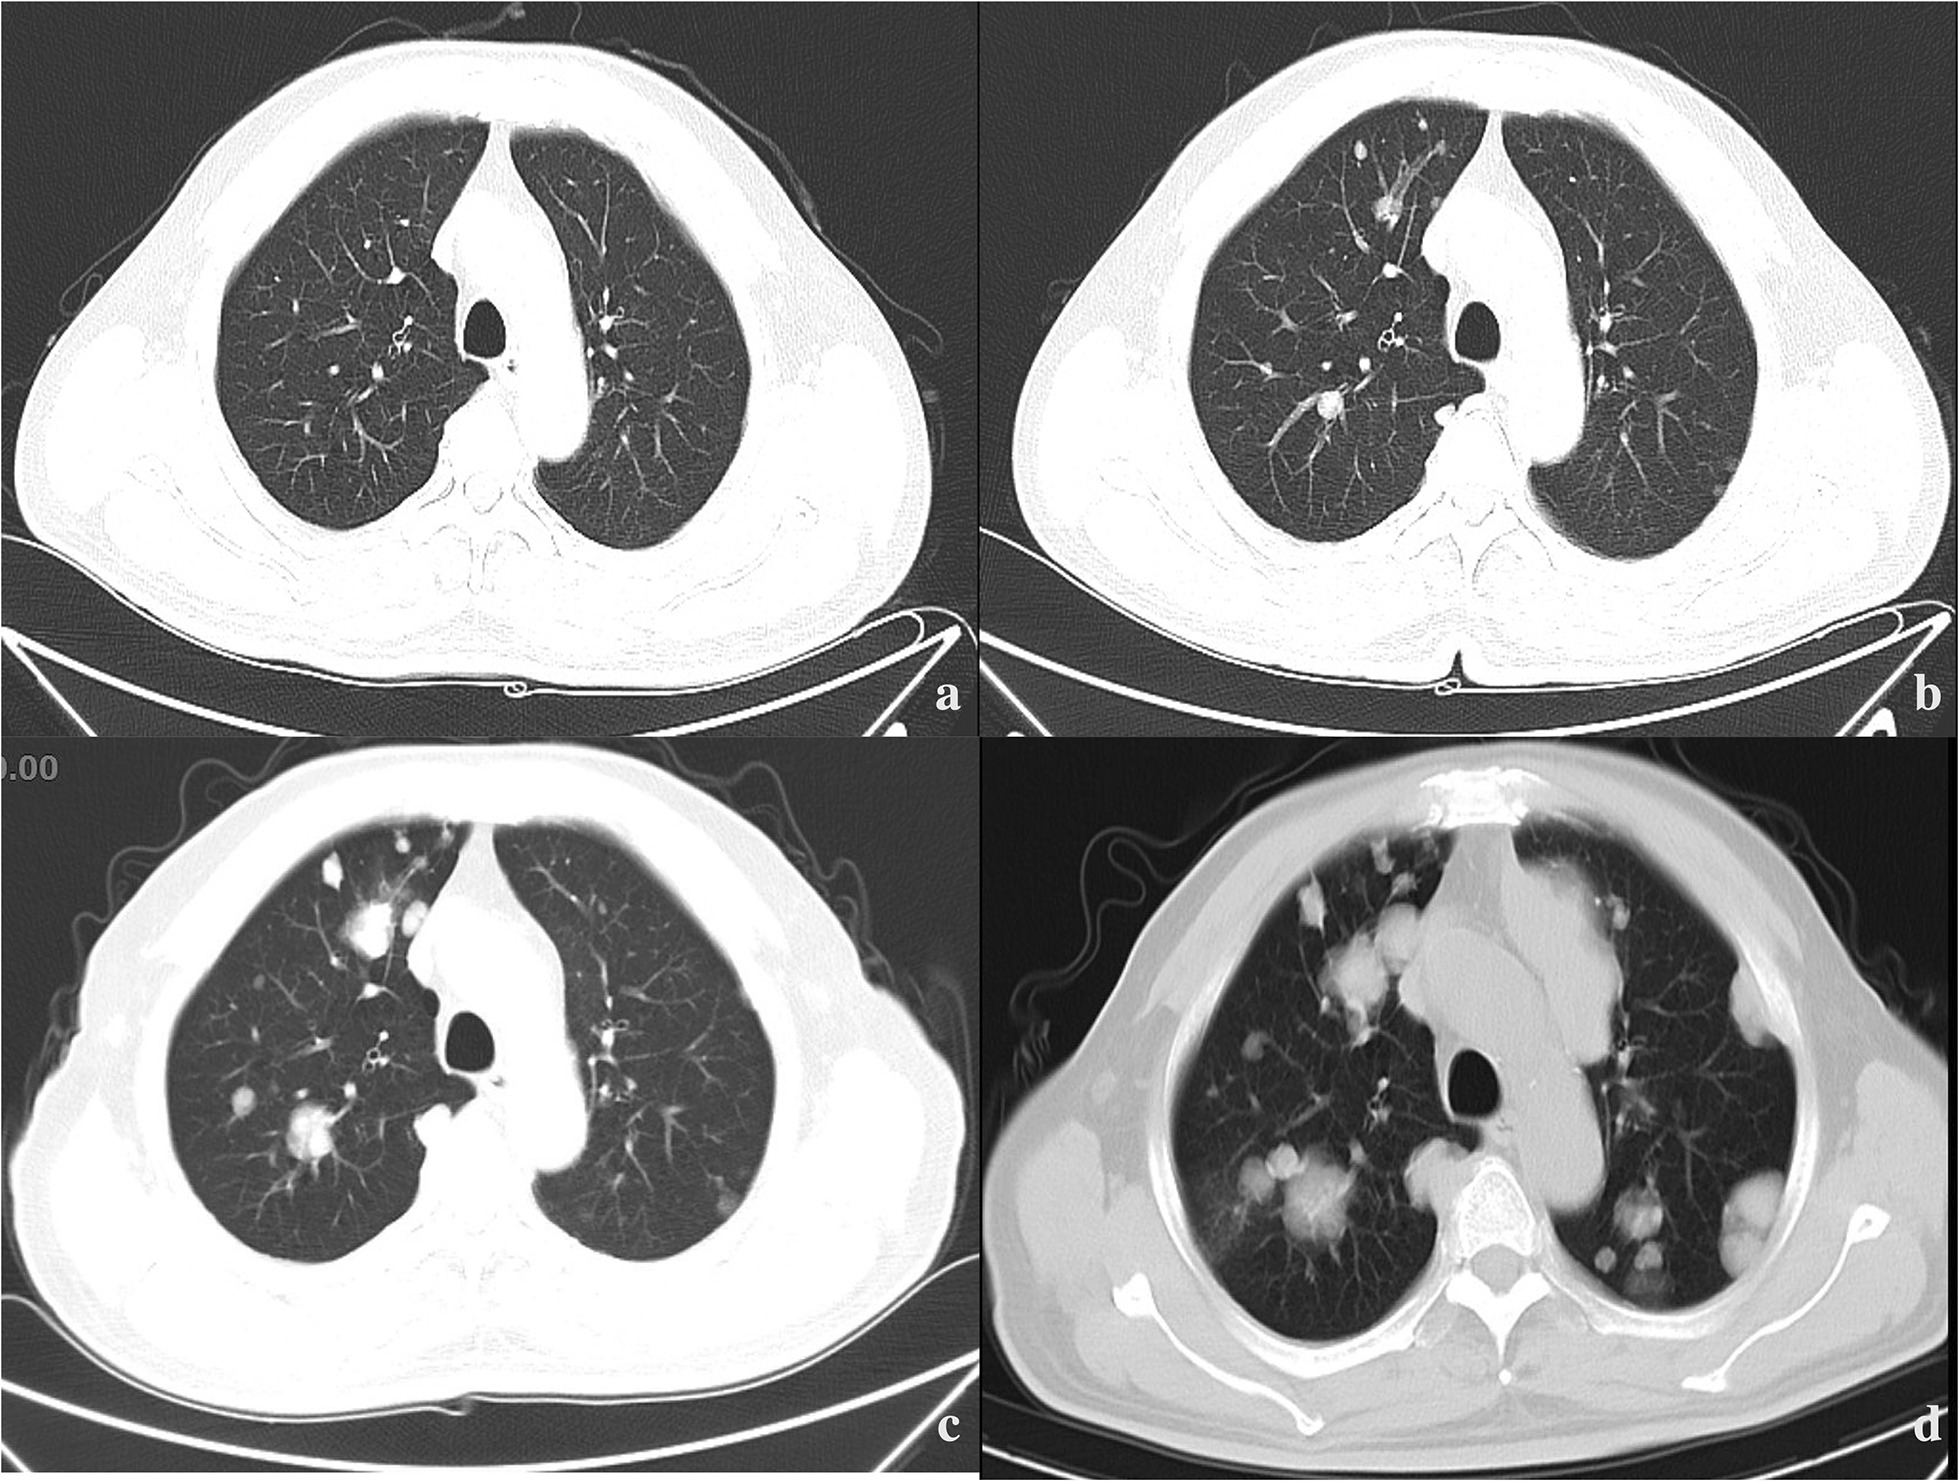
CT images of pulmonary: a showed no lung metastases when the patient was diagnosed on March 2021; b showed multiple metastases located in lungs on June 2021, after orchiectomy and 2 cycles of chemotherapy; c showed progressed lung metastases on August 2021, after 2 cycles of second- or third-line chemotherapy; d showed recently progressed lung metastases on January 2022
